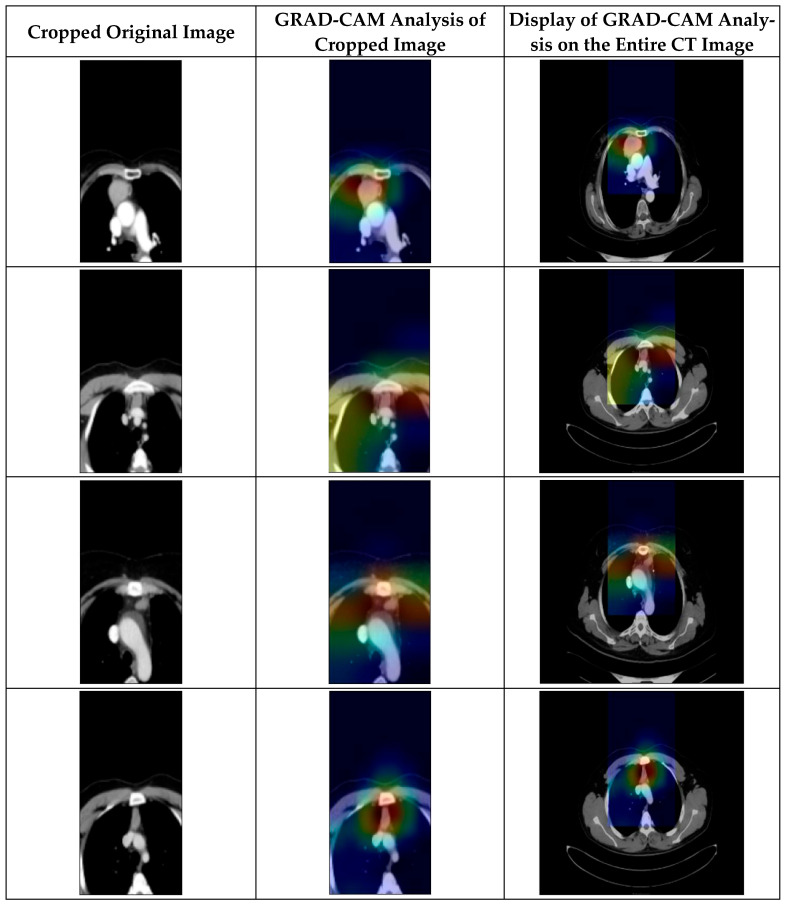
GRAD-CAM analysis of CT images of samples with thymoma tumors.
